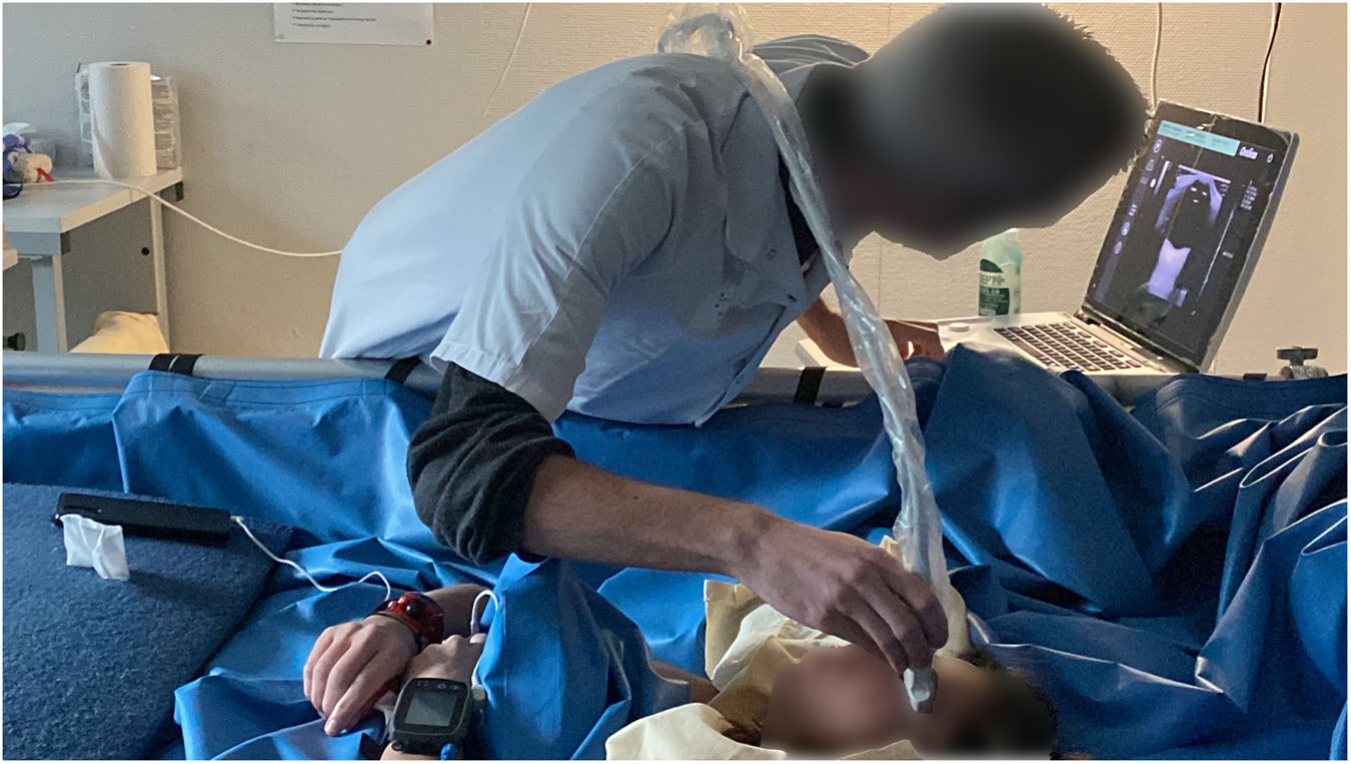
Optic Nerve Sheath Diameter measurement by ultrasound in Dry Immersion (Credit: MEDES).
